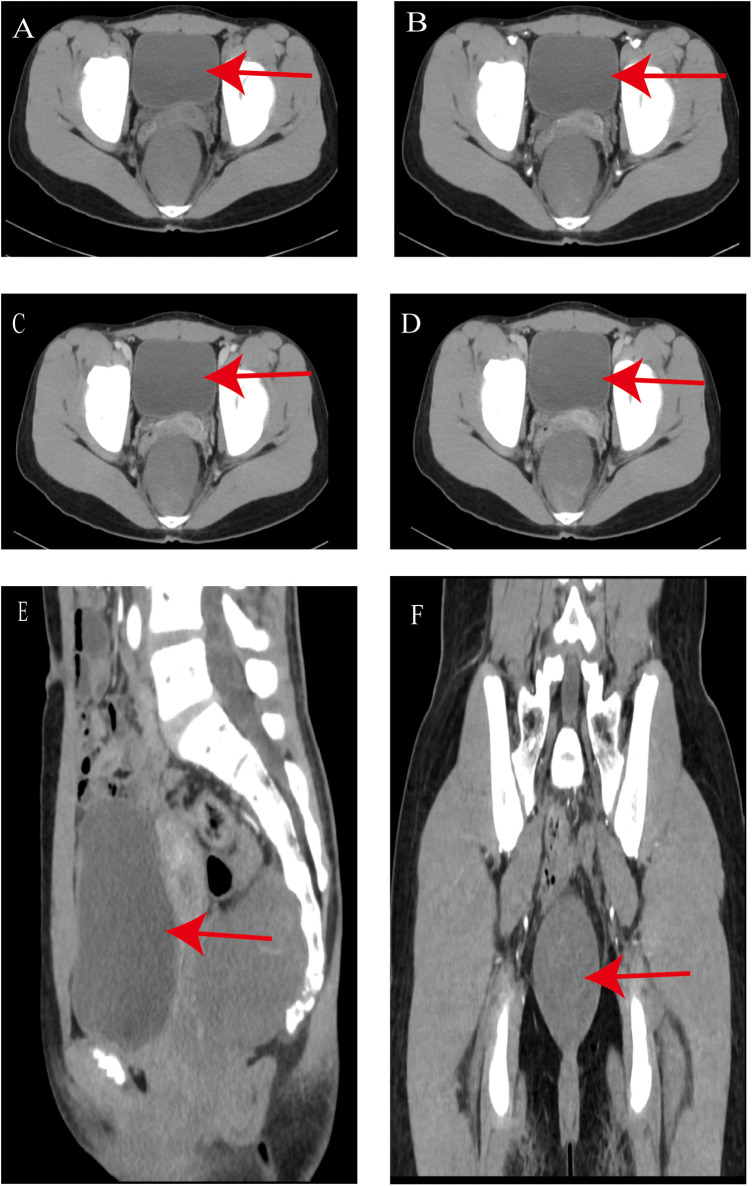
Preoperative CT findings (May 25, 2022) (A) Non-contrast (CT value approx. 27 HU); (B) Arterial phase (CT value approx. 30 HU); (C) Venous phase (CT value approx. 32 HU); (D) Delayed phase (CT value approx. 35 HU) Mild enhancement is observed; (E) Sagittal view; (F) Coronal view.
